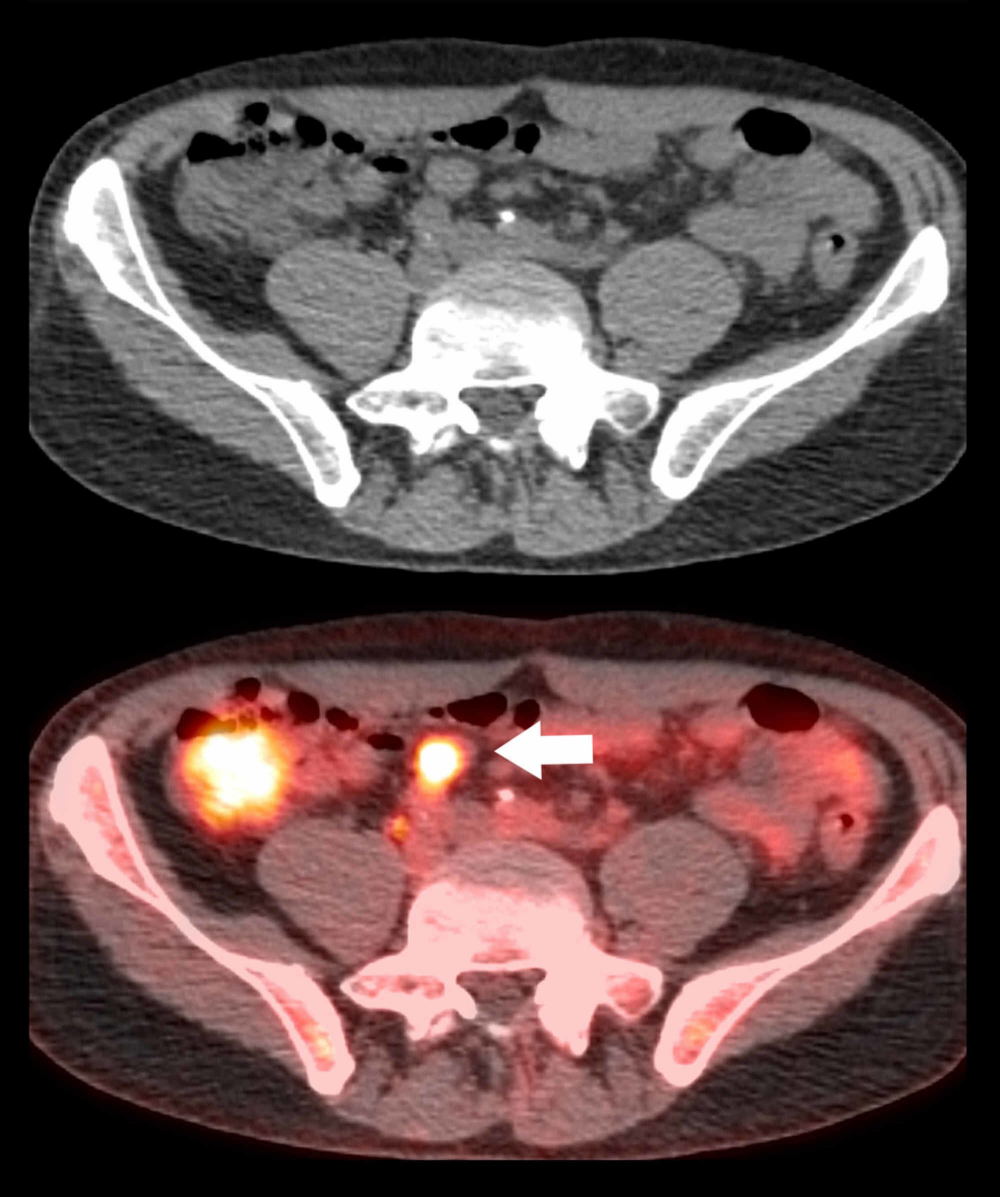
Axial CT and PET-CT fusion images. Axial CT and PET-CT fusion images show an enlarged hypermetabolic lymph node within the mesenteric region of the pelvis (wide arrow) with standardized uptake value (SUV) 6.8 which represents recurrent lymphoma.
